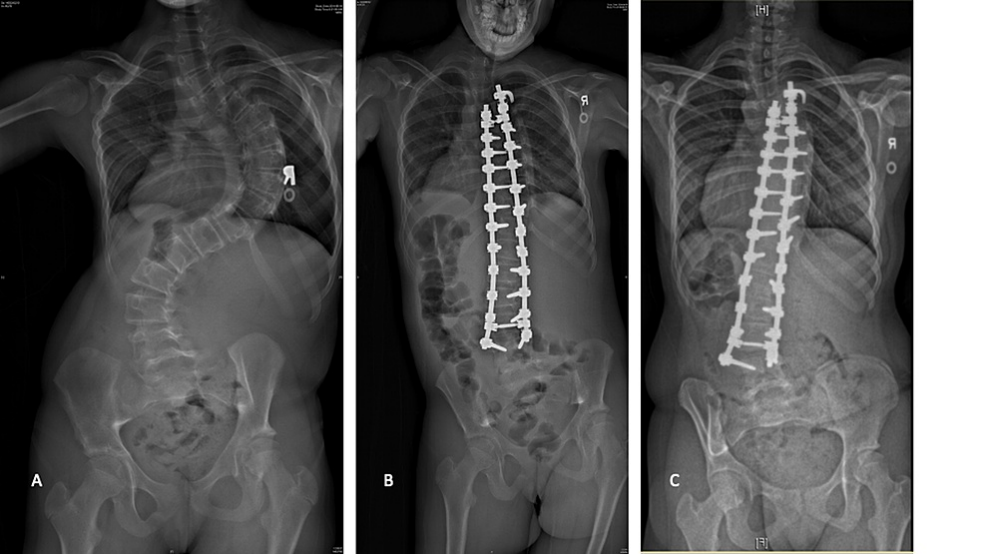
The patient diagnosed with adolescent idiopathic scoliosis showing pullout of the distal pedicle screws associated with non-union. The patient was diagnosed with adolescent idiopathic scoliosis showing pullout of the distal pedicle screws associated with non-union. (A) Pre-operative AP radiograph; (B) immediate post-operative AP radiograph; (C) AP radiograph showing pullout of the left L4 pedicle screw.
